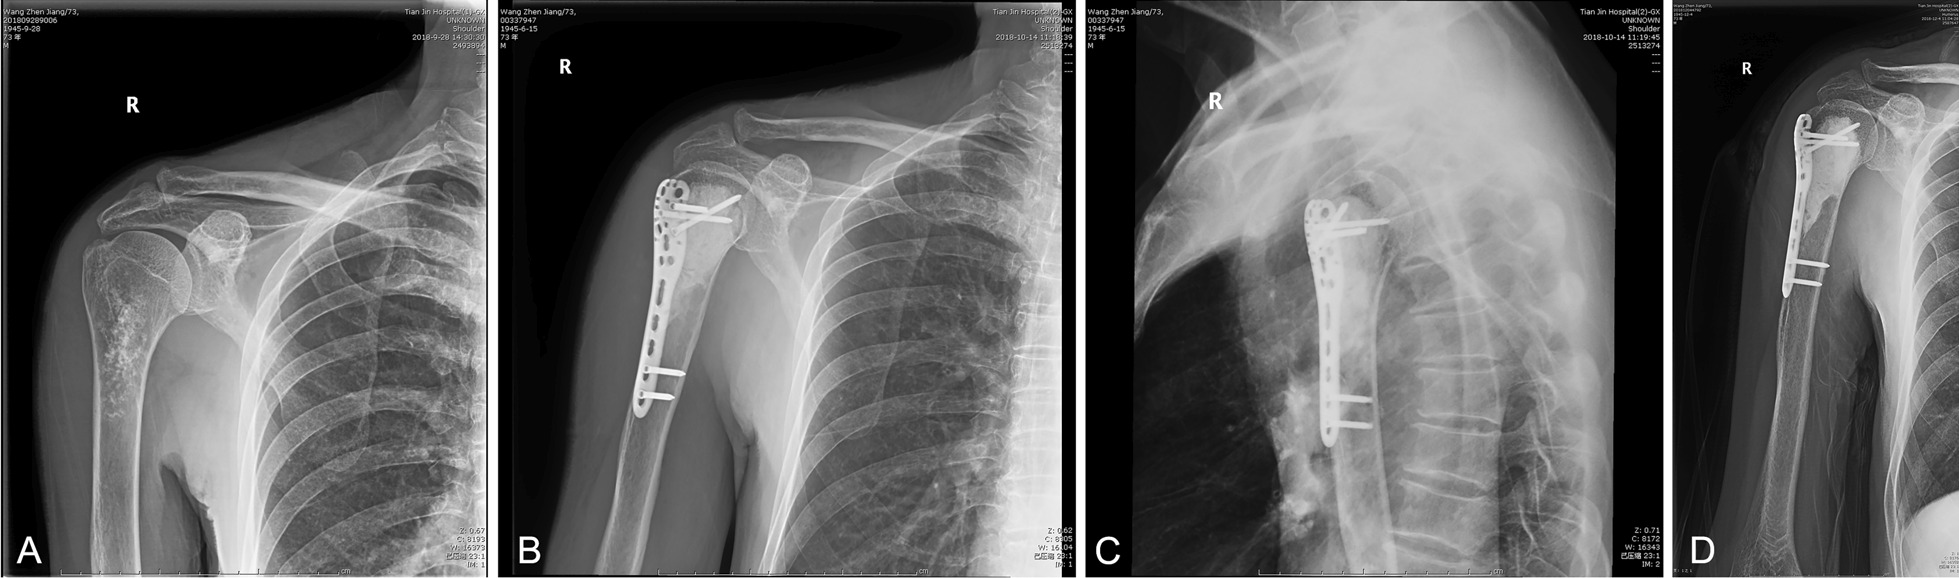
A 73-year-old male with enchondroma on the proximal humerus, who was operated with tumor resection followed by microwave-induced hyperthermia in situ and internal fixation with bone cement. A: anteroposterior (AP) radiograph of the right shoulder showed sand-like calcification lesion of the upper humerus. B & C: the AP and lateral radiographs of the right shoulder at 3 days after operation. D: the AP radiograph of the right shoulder at 2 months after operation
